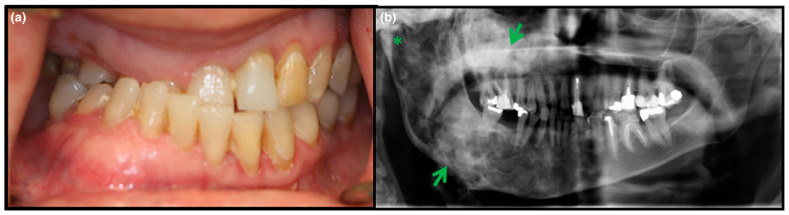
A patient with craniofacial FD. (a) Significant malocclusion can be observed with dental crowding and malrotation of the teeth. (b) Radiograph of the same patient revealing maxillary and mandibular FD (green arrows). Involvement of the mandibular condyle can also be seen (asterisk). Adapted with permission from Ref. [41]. 2017, *Oral Diseases*.
